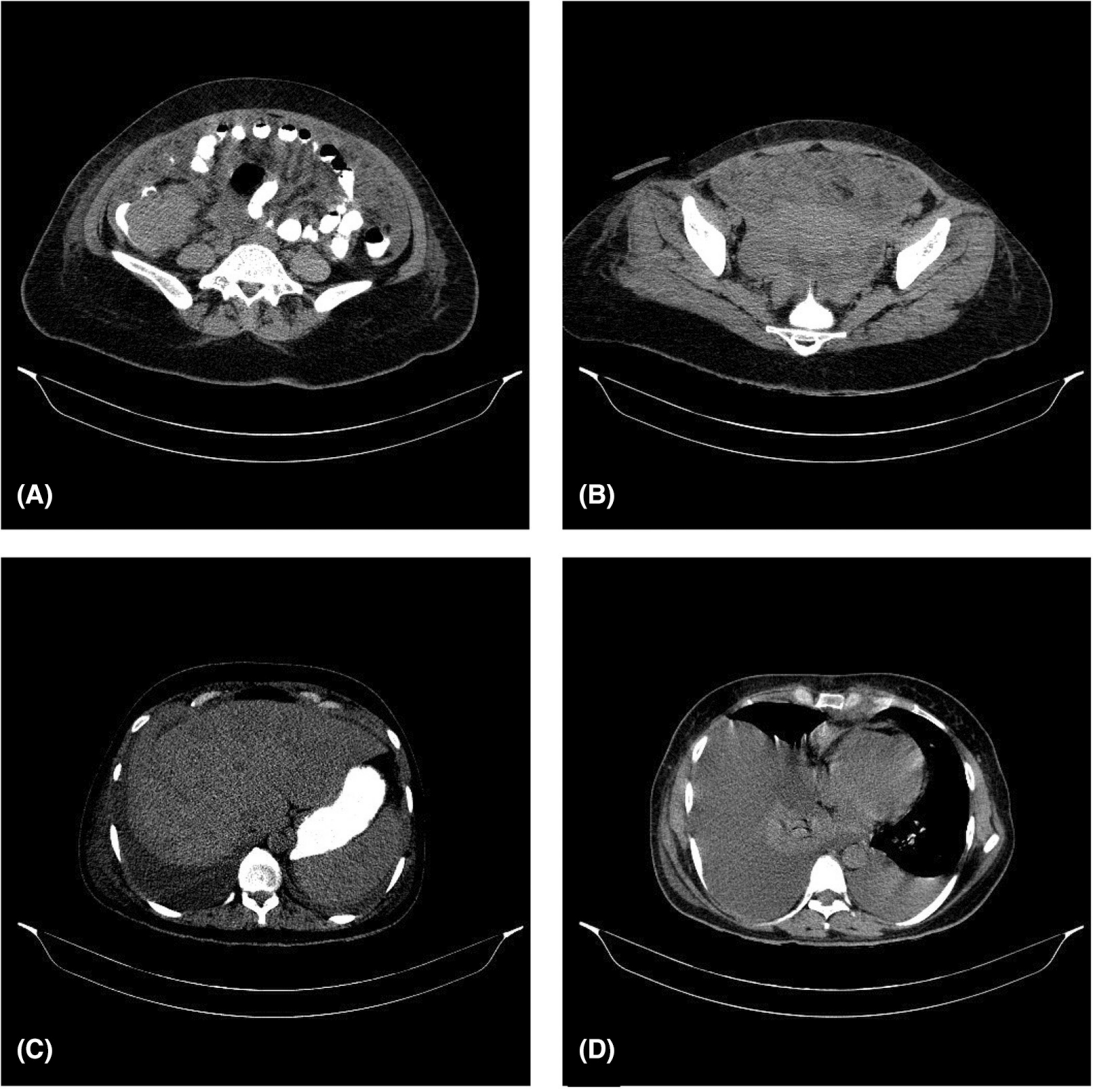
Thoracoabdominal CT scan with oral contrast showing: (A) cecal mass, (B) omental infiltration, (C) liver lesions, and (D) pleural effusion.
